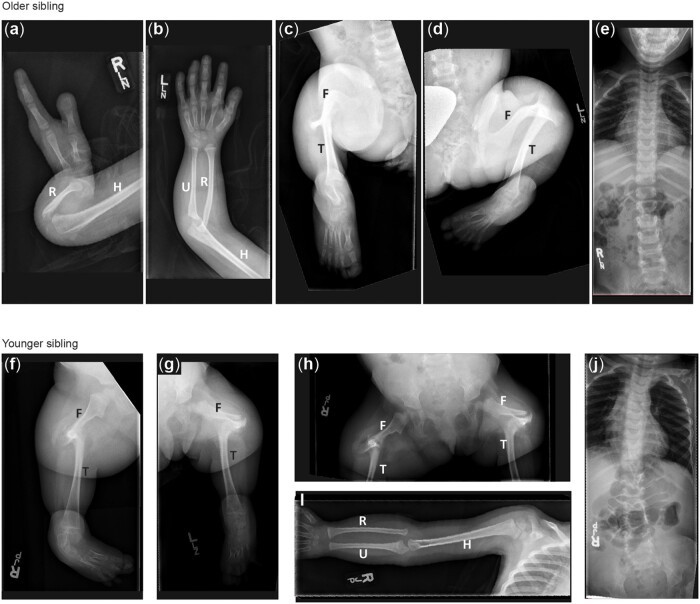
Radiographic features of siblings with a VUS in *HNRNPUL1*. a–e) X-ray images of older sibling. Right arm showing short humerus and absent ulna with 2 fixed in extension digits of the right hand (a). Left arm showing short humerus and normal upper arm (b). Right (c) and Left (d) legs showing mid-shaft femoral pseudoarthroses, fused tibia to the femoral condyles, absent fibulas, and abnormal toes. f–j) X-ray images of the younger sibling Right (f) and Left (g) legs showing bilateral fibular agenesis, short and bowed femurs, and 4 metarsals and tarsals (h). Right arm (i) showing normal upper limb development.
